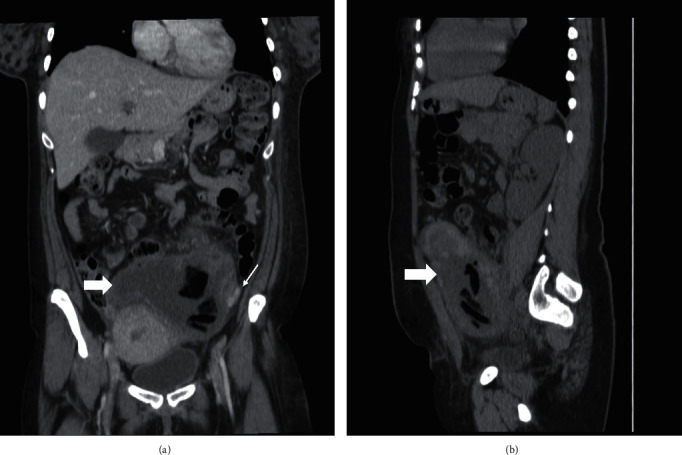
Computed tomography images of the left ovarian cyst (thick arrow) (3 months later). Large heteroechoic cystic lesion that drained to the left enlarged ovarian vein (thin arrow) with several bizarre shapes, low density lesions inside the left ovary, as shown on (a) coronal section and (b) sagittal section.
